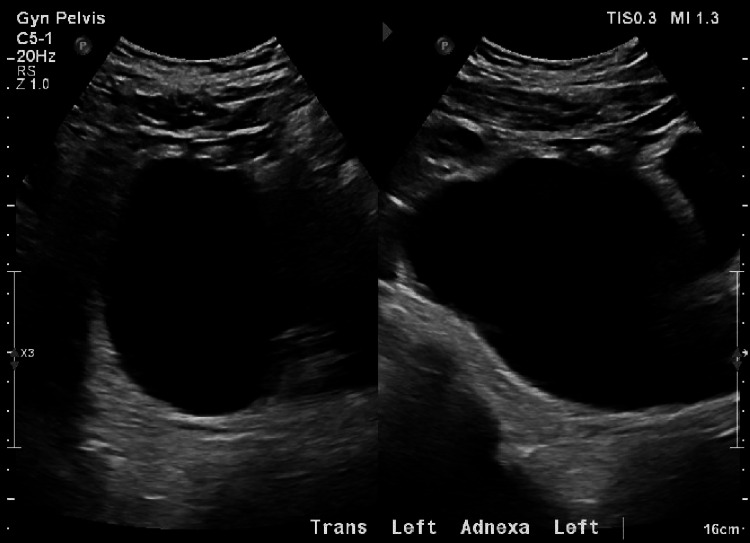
Left Ovarian Cyst on Transvaginal Pelvic Ultrasound
